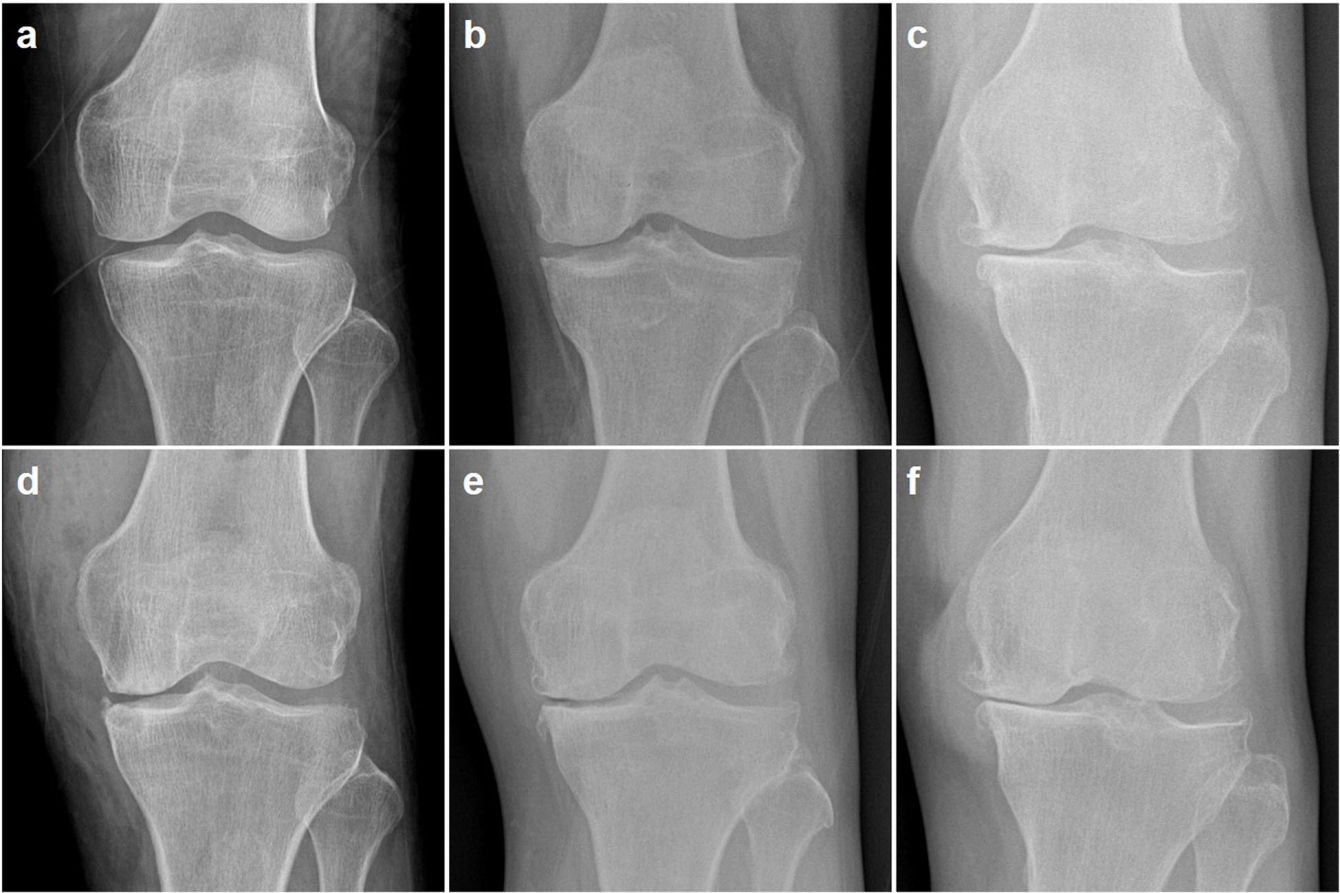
Radiographs showing the weight-bearing anterior-posterior (AP) views at baseline (a–c) and 1 year after injection (d–f). Radiographs of a 59-year-old man revealing a progression of knee osteoarthritis (OA) from Kellgren-Lawrence (K-L) grade 1 at baseline (a) to K-L grade 2 at 1 year after hyaluronic acid (HA) injection (d). Radiographs of a 64-year-old woman demonstrating an advancement of knee OA from K-L grade 2 at baseline (b) to K-L grade 3 at 1 year after mesenchymal stem cell (MSC) injection (e). Radiographs of a 62-year-old woman depicting a deterioration of knee OA from K-L grade 3 at baseline (c) deteriorating to K-L grade 4 at 1 year after HA injection (f)
